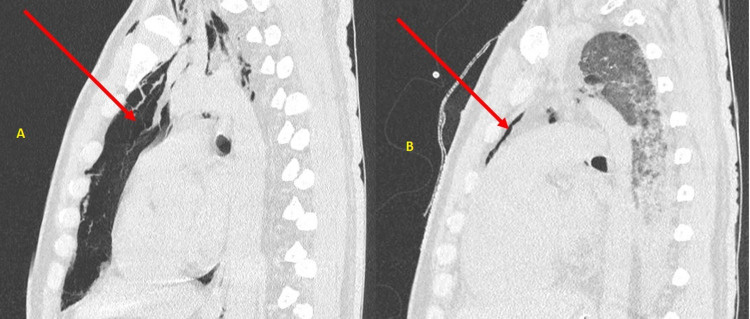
(A) High-resolution computed tomography of the thorax – sagittal section showing pneumomediastinum (red arrow). (B) High-resolution computed tomography of the thorax – sagittal section showing reduction in the size of the pneumomediastinum following pigtail insertion (red arrow).
